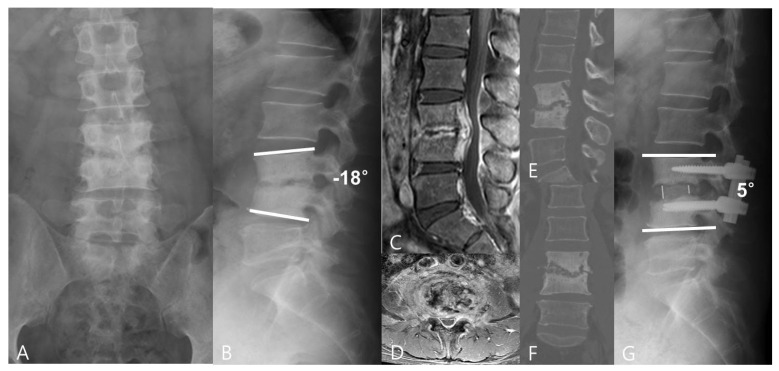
A 44−year−old man had lower back pain for more than 2 months. (A,B) The preoperative anteroposterior and lateral radiographs showed L3/4 intervertebral space narrowing and regional kyphosis. (C,D) Preoperative CT and (E,F) MRI images revealed L3/4 pyogenic spondylodiscitis with endplate destruction. A minimally invasive oblique lumbar interbody fusion using PEEK cages with an autologous bone graft was performed. (G) Plain lateral radiographs at the last follow-up showed L3/4 interbody fusion and good correction of regional kyphosis.
